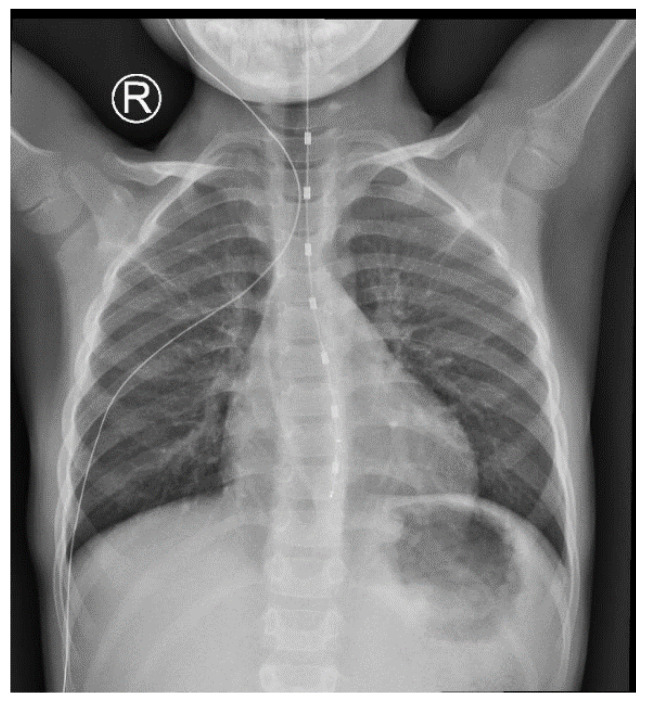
Chest radiograph from one of our patients for verification of esophageal impedance–pH catheter position. The catheter is visualized along the esophagus with electrodes in appropriate position. Lung fields and mediastinal structures appear within normal limits. The R sign indicated the right side of the patient.
